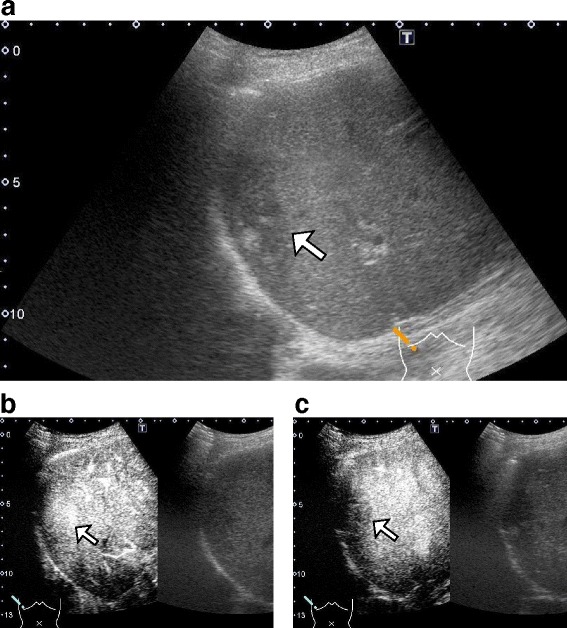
Enhanced ultrasonography using perfluorobutane microbubble. An irregular-margin of low echoic mass depicted by B-mode image (a). The lesion infused by microbubble at 10 s after contrast injection (b). Defected microbubble enhancement at 20 s (c)
